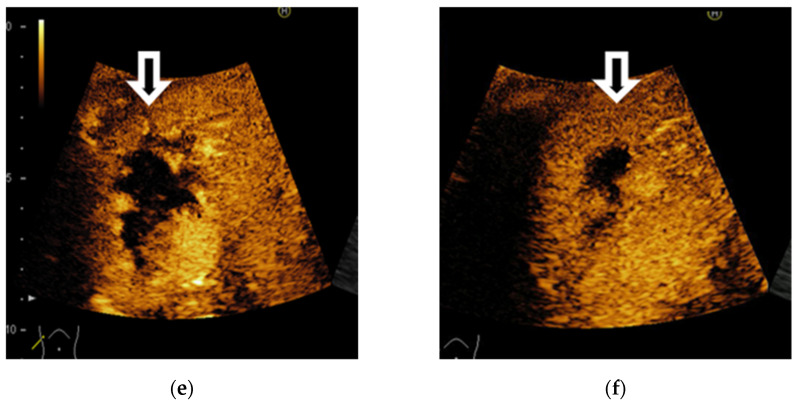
A case of focal liver lesions detected in a 68-year-old man with liver cirrhosis, characterised as LI-RADS-1 on CEUS. On B mode ultrasound is observed a hyperechoic inhomogeneous liver, nodular liver surface and a hypoechoic, inhomogeneous FLL in the right liver lobe (a). On CEUS, the liver lesion shows a typical early, peripheral, globular enhancement (b,c) and centripetal fill-in (d,e). In the late phase, incomplete enhancement is noticed (f).
